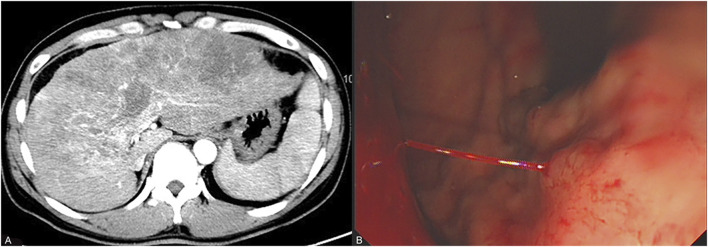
Results of the patient’s enhanced CT scan and gastroscopy. (A) represents the enhanced CT showing that the patient is in the stages of cirrhosis and advanced liver cancer 2 months before admission. (B) represents the emergency gastroscopy showing that the patient is experiencing acute gastric variceal bleeding 1 day before admission.
